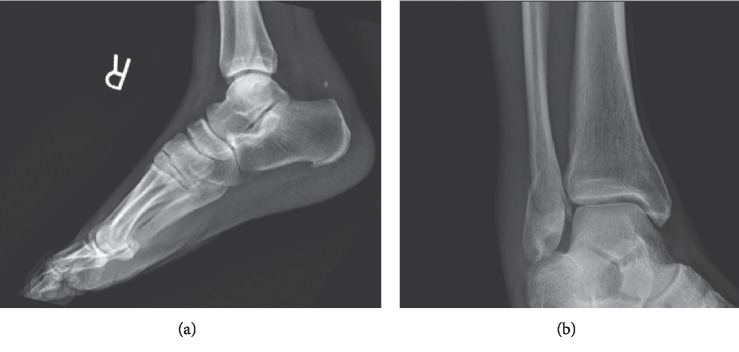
Plain radiographs: (a) lateral view and (b) mortise view.
